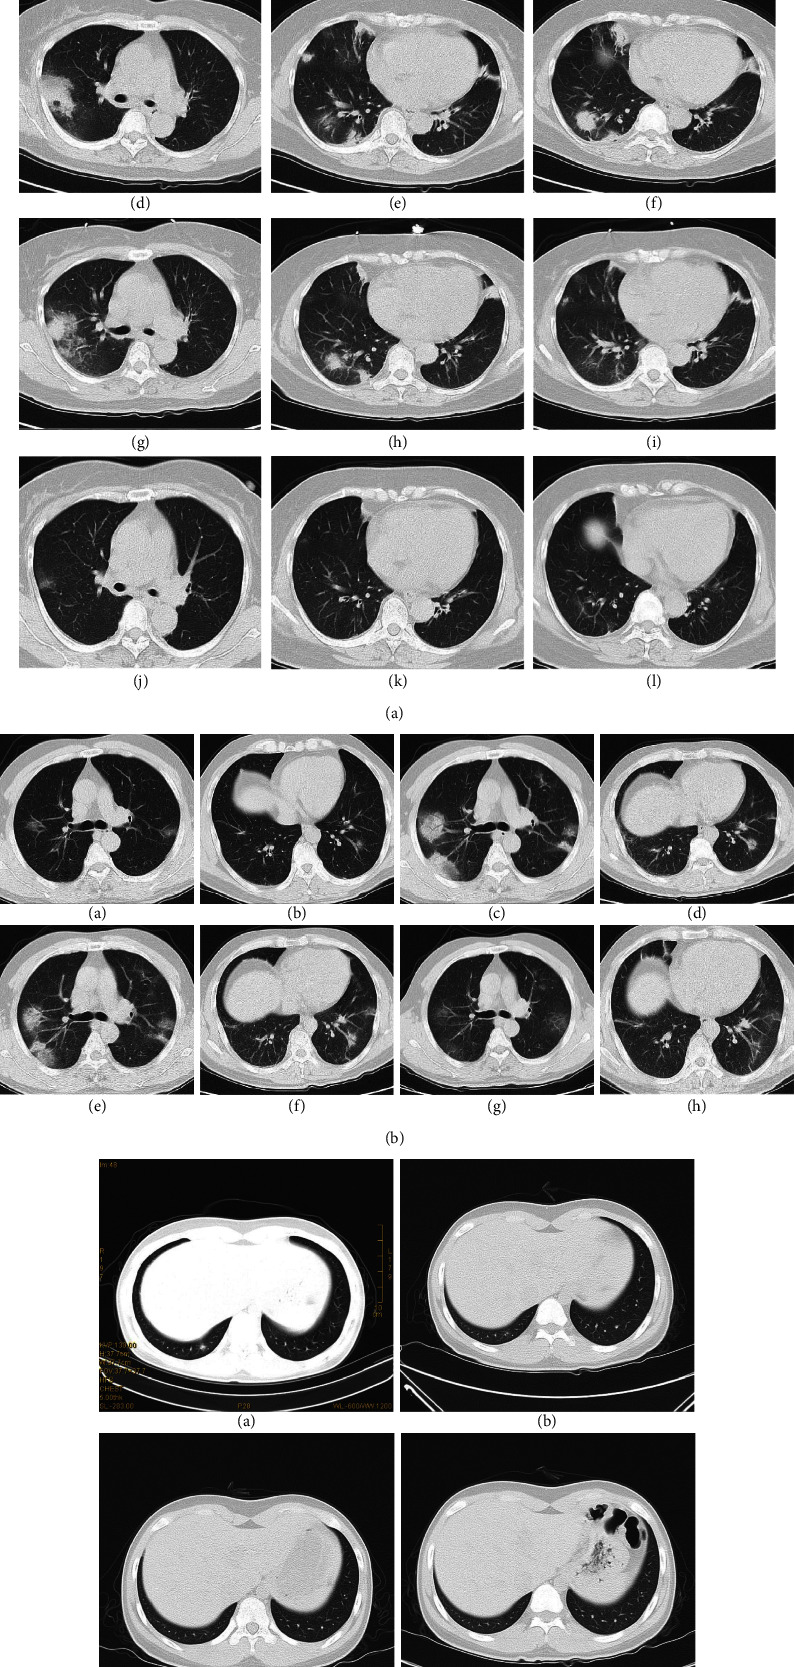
(a) Computed tomography imaging of an asymptomatic carrier who developed symptoms at a later stage. A 57-year-old woman who had hypertension for five years had close contact with a confirmed COVID-19 patient (her husband). (A) The first computed tomography imaging scan showed an area of consolidation in the right middle lobe. (B and C) Seven days later, the woman developed a slight cough; computed tomography imaging showed that the area of consolidation was enlarged in the right middle lobe. (D–F) At 11 days, new lesions developed in both the upper and lower lobes. (G–I) In the early improvement stage at 13 days, lesions in the bilateral lung area shrank and decreased in density. (J–L) The lesion in the left lung had disappeared, while the right lung lesions had been markedly absorbed. (b) Computed tomography imaging of a symptomatic COVID-19 patient. A 46-year-old man who lived in Wuhan had an intermittent cough and tested positive for viral nucleic acid. (A and B) On the day of admission, multiple patches of ground-glass opacity were observed in both upper lobes and the left lower lobe. (C and D) Four days later, computed tomography imaging showed that the lesion in both upper lobes was enlarged, and the ground-glass opacity had transformed into ground-glass opacity mixed with consolidation. An air bronchogram was observed in the right upper lobe, and new lesions appeared in both upper lobes. (E and F) In the early improvement stage, the lesions in both upper lobes shrank and reflected decreased density. (G and H) In the late improvement stage, the lesions in both upper lobes had been markedly absorbed with only patchy ground-glass opacity remaining. (c) Computed tomography imaging of a special case without symptoms (an asymptomatic carrier). A 16-year-old boy was isolated because his parents were confirmed symptomatic COVID-19 patients. (A) The first computed tomography imaging scan revealed a small high-density lesion (arrow) in the right lower lung lobe. (B) Four days later, the lesion in the right lower lobe had undergone marked shrinking (arrow). (C) At the six-day follow-up, the lesion had further shrunk and became a lesion of only 0.5 cm in diameter (arrow). (D) At the 11-day follow-up, the lesion had completely disappeared.
